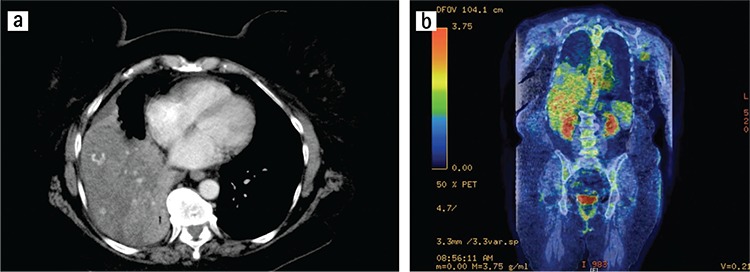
Axial CT and coronal PET-CT slices showing a huge malignant solitary fibrous tumor mass in the lower lobe of right lung.
